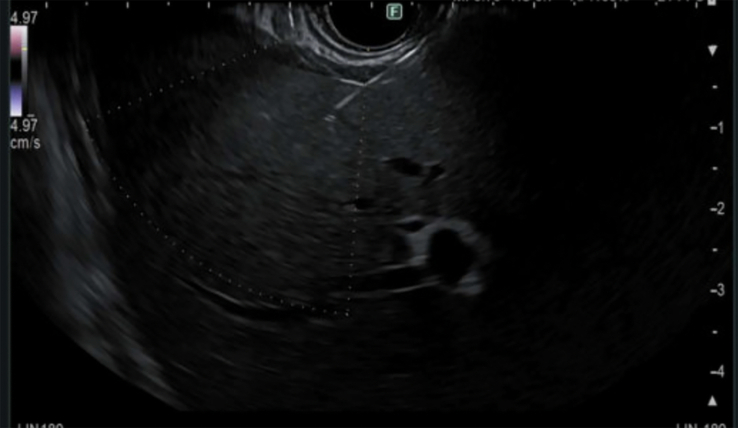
EUS-guided parenchymal liver biopsy using a 22-G core needle. Abbreviation: EUS, endoscopic ultrasound.
